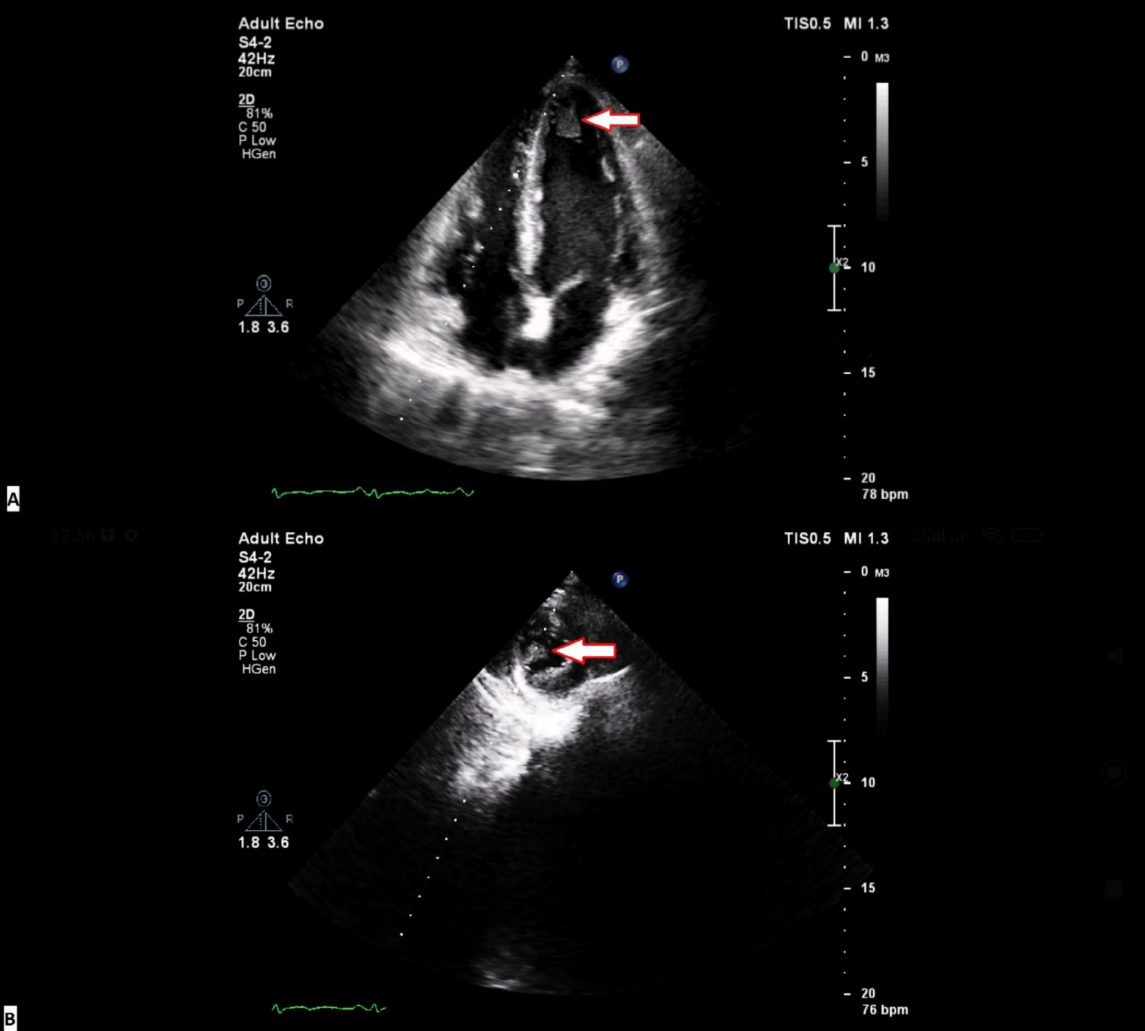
Left ventricular mass on transthoracic echocardiography. (A) Apical four‐chamber (A4C) view. (B) Left ventricular short axis view.
